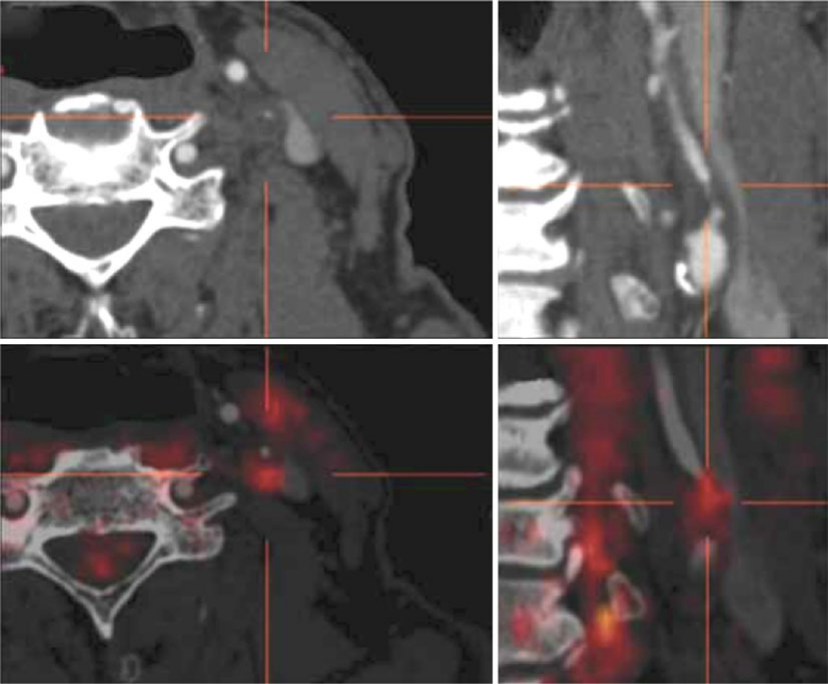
Detection of inflamed plaque in a symptomatic patient a significantly stenotic left internal carotid artery. In transverse and coronal contrast-enhanced CT images (*top row*), there is evidence for significant obliteration of the lumen with little calcification on CT. Hybrid PET/CT images provide evidence for increased [18F]fluorodeoxyglucose at
